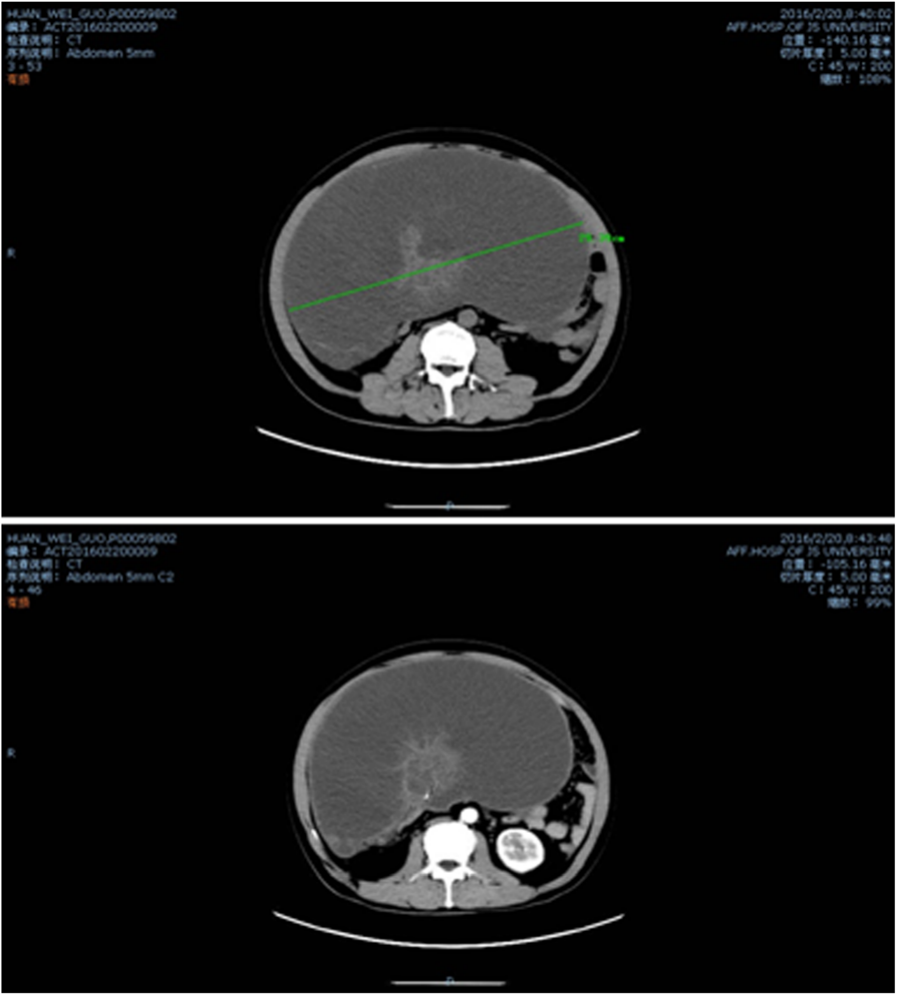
An abdomen computed tomography scan revealed a giant cystic mass in the abdominal-pelvic cavity
